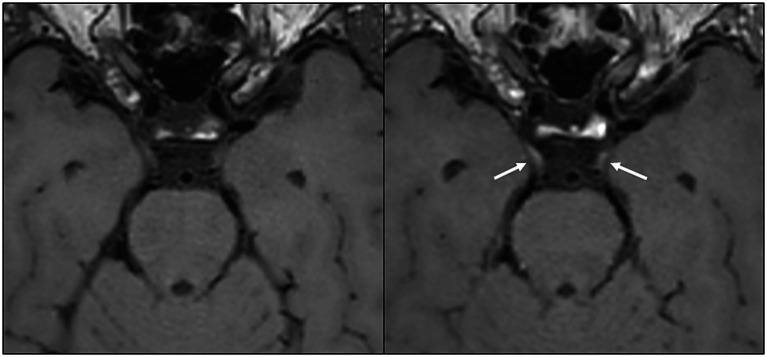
Axial image reconstruction from a black-blood T1 space sequence before (left) and after (right) contrast administration, showing bilateral contrast enhancement in the cisternal portion of the oculomotor nerve (arrows). This MRI finding was present 13.5 months after the onset of COVID-19 in a middle-aged woman who developed a new-onset, persistent post-infectious headache.
